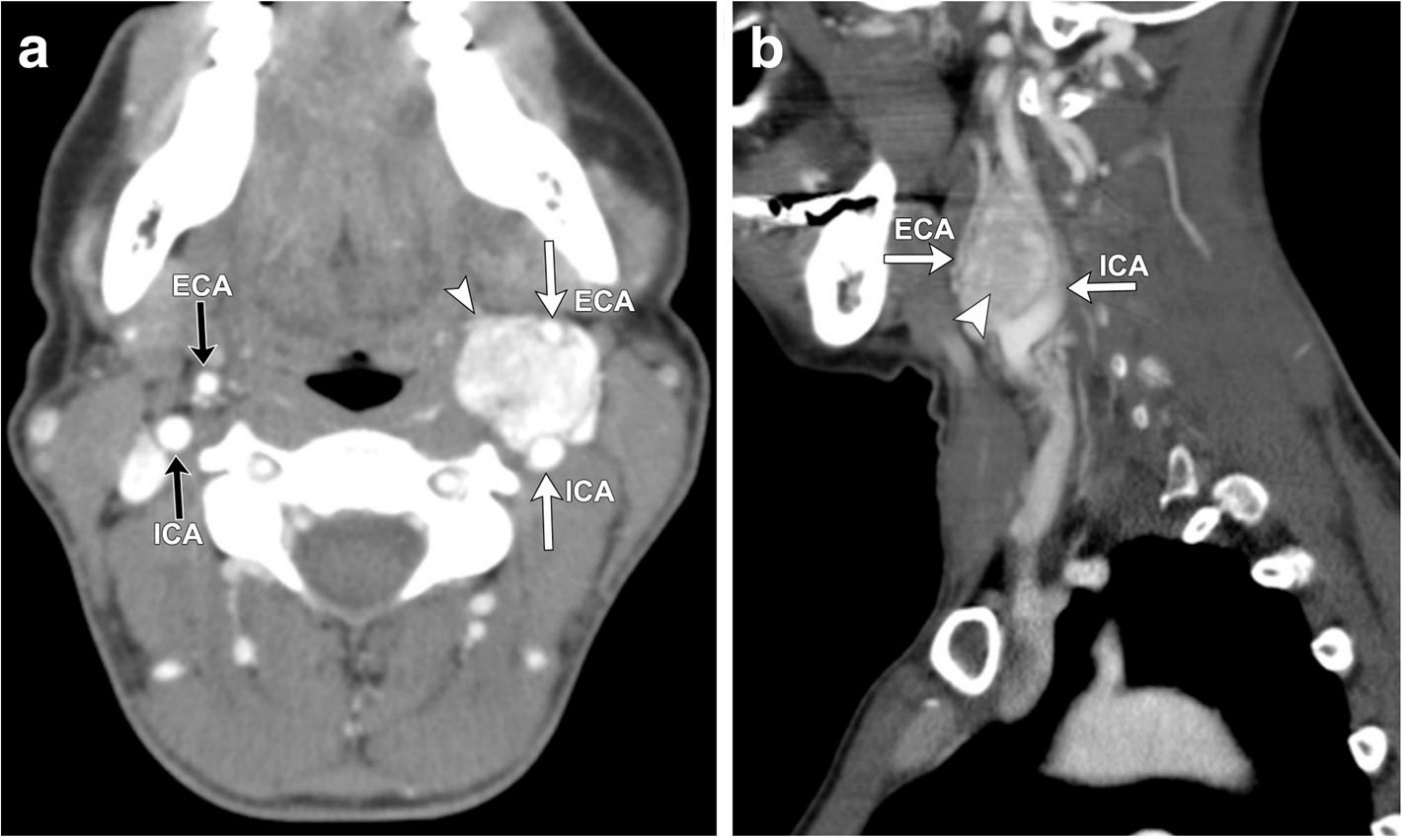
Axial (a) and sagittal (b) contrast-enhanced CT of the neck demonstrates a well-defined, avidly enhancing soft tissue mass (white arrowheads) within the left carotid space. The mass splays the proximal external and internal carotid arteries (white arrows) at the level of the carotid bifurcation, characteristic of a carotid body tumor (as opposed to a glomus vagale, which typically displaces the ICA and ECA together). The mass encases less than 50% of the left ICA. Note the normal relation of the right ECA and ICA on the contralateral side of the neck (black arrows)
